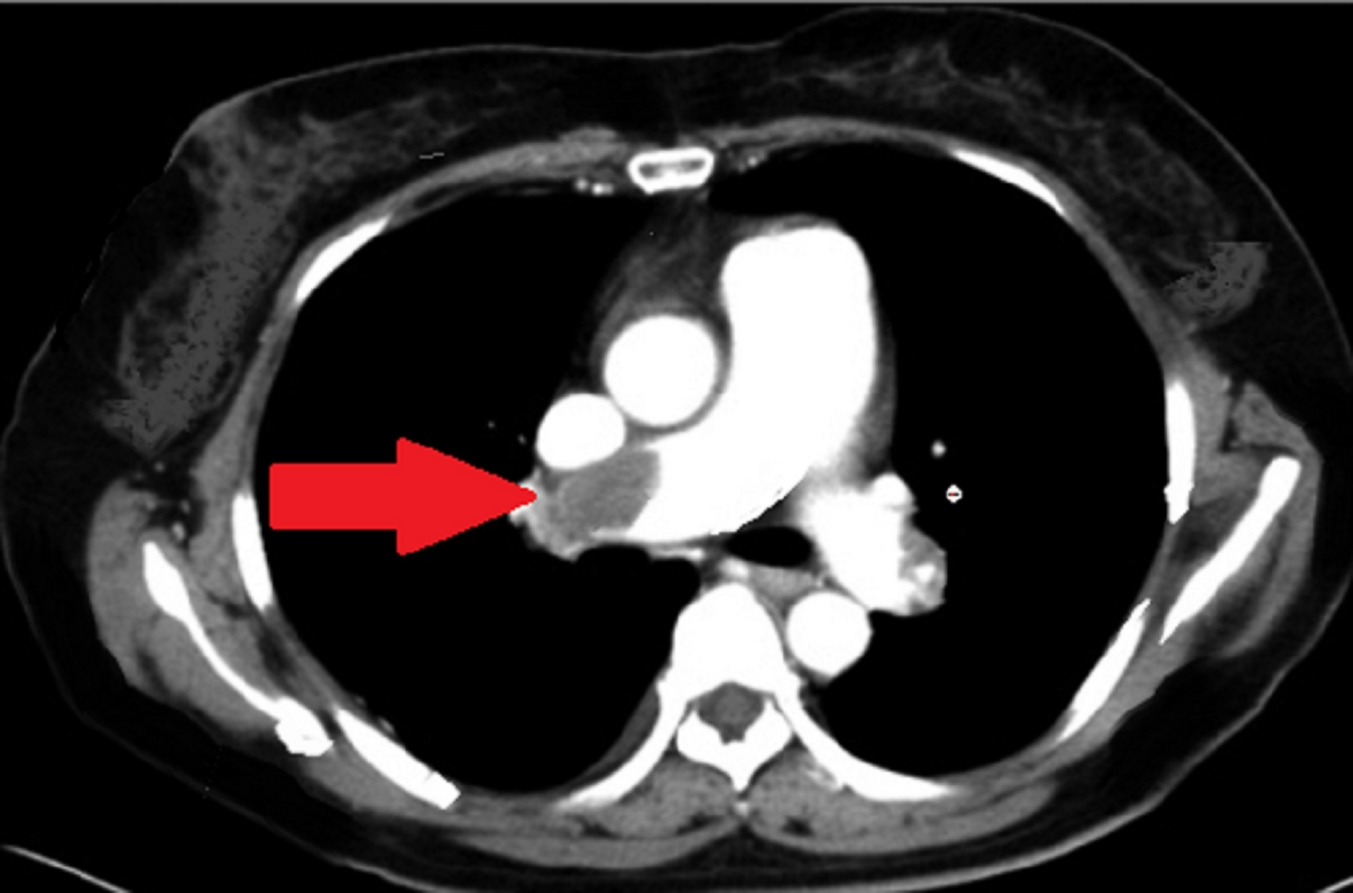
CT pulmonary angiogram showing thrombus in the right pulmonary artery CT: computed tomography
